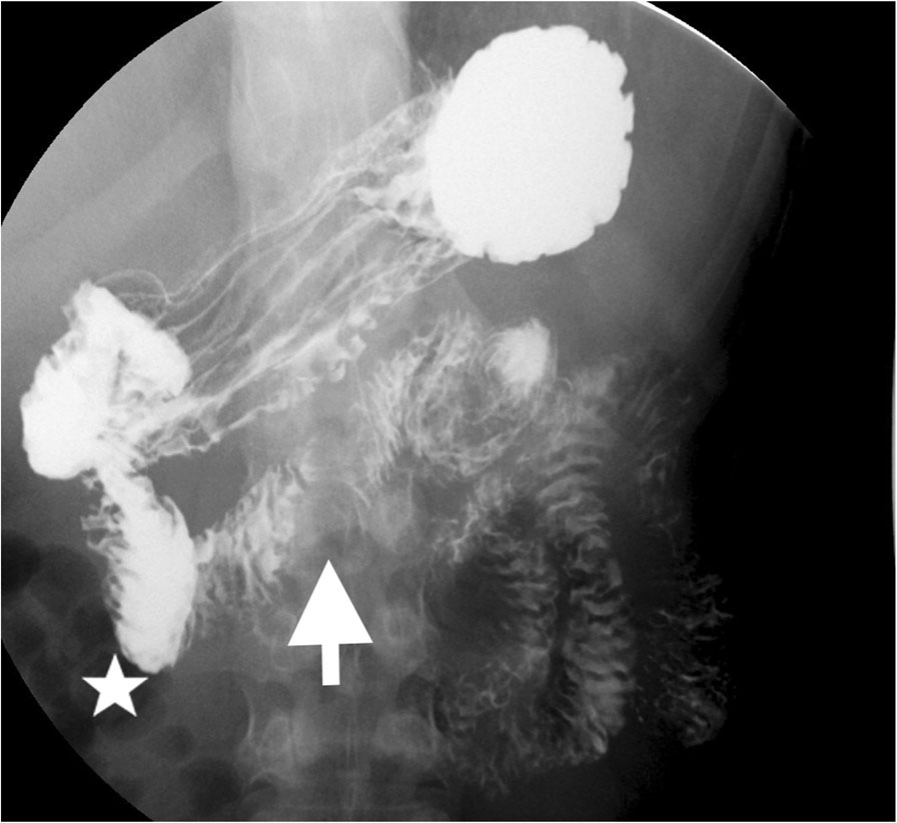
Upper gastrointestinal series/Upper gastrointestinal double-contrast radiograph. A vertical band of extrinsic compression (arrow) on the mid transverse part of duodenum compressed by SMA with proximal duodenal dilatation (star)
